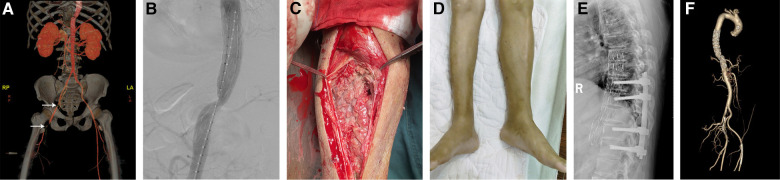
Filling defect at the right external iliac artery and femoral artery bifurcation with severe luminal stenosis (white arrows) (A). Angiogram obtained on completion of the procedure showing adequate placement of the stent graft with no endoleak or kink (B). Thirteen days after the right lower leg fasciotomy, there was still extensive necrosis in the posterior leg muscles (C). The circumference of the right calf was smaller than that of the left calf at 7 months after flap reconstruction surgery (D). A lateral radiograph obtained after 1 year of follow-up shows the aortic stent graft *in situ* and stabilization of the vertebral fracture at T10 (E). A repeated CTA performed 1 year of follow-up showed the stent without endoleak (F).
